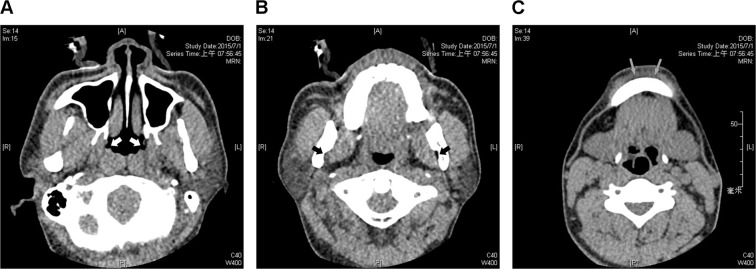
Radiographic landmarks of three representative parapharyngeal fat pad areas in axial images. The nasopharyngeal level of parapharyngeal fat pad was defined as where the Eustachian tube orifices (white arrows) are located (A). The oropharyngeal level was defined as where the mandibular foramens (black arrows) are located (B). The subglosso-supraglottic level was defined as where the mental tubercles (grey arrows) are located (C).
